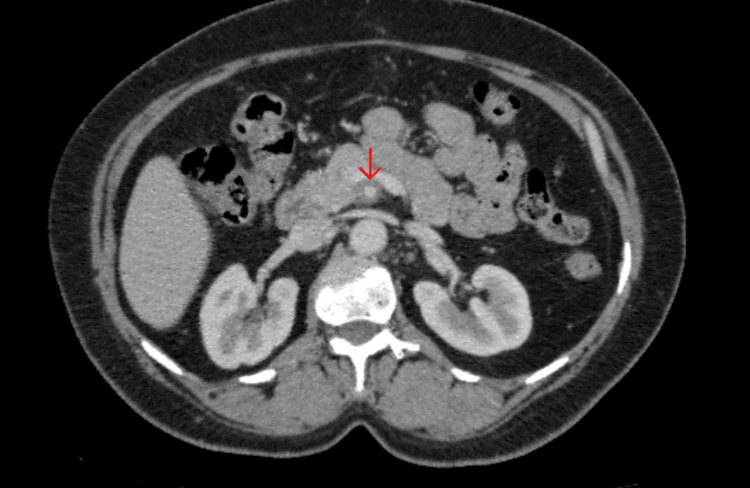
CT scan at nine month follow-up. The CT scan is showing an almost complete regression of the mass. Red arrow pointing to the mass.
